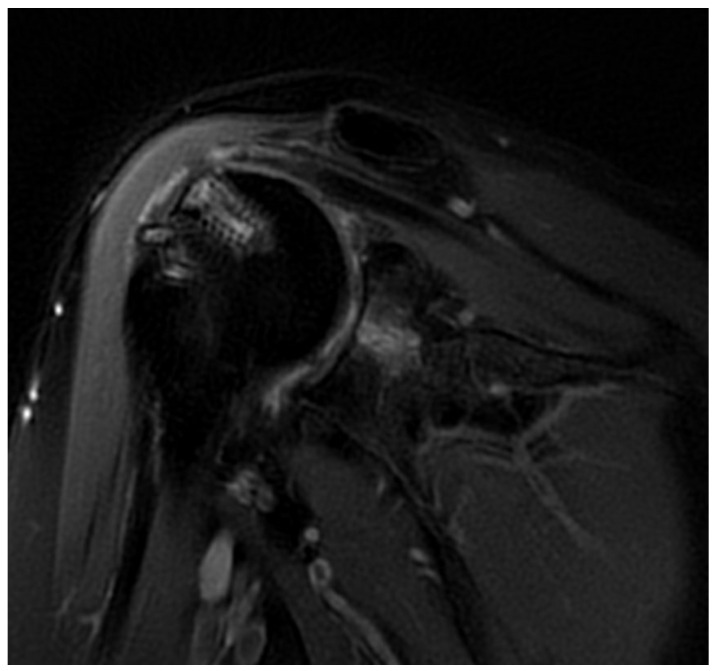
T2-weighted sagittal MRI of a shoulder following successful healing of a supraspinatus tear with DBM, PRP, and cBMA augmentation.
